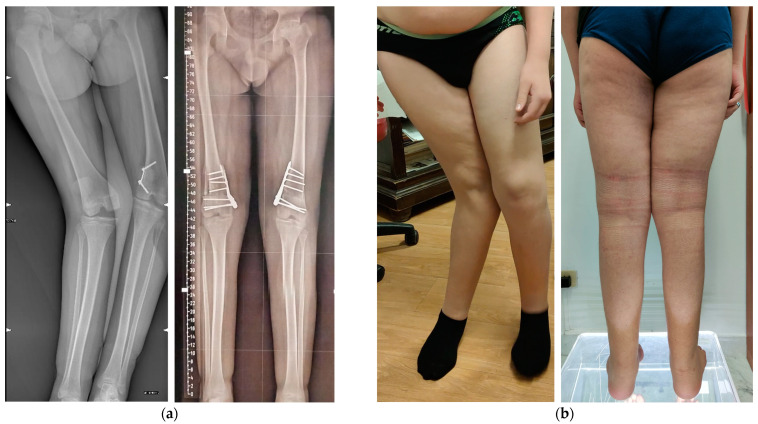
(a) Preoperative and postoperative (+6 month) radiographs; (b) Clinical pictures before and after surgery.
